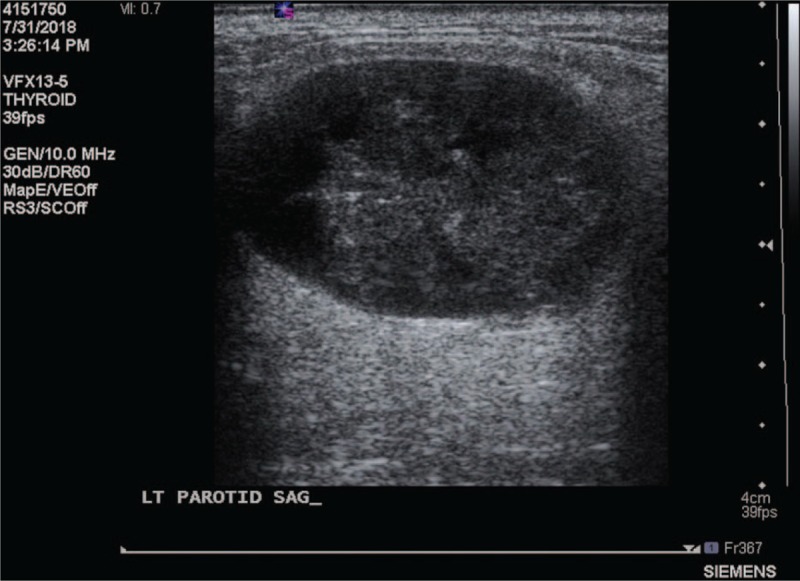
Target ultrasonography of the left parotid gland in sagittal view revealing a nonhomogeneous hypoechoic tumor with an internal hyperechoic component.
